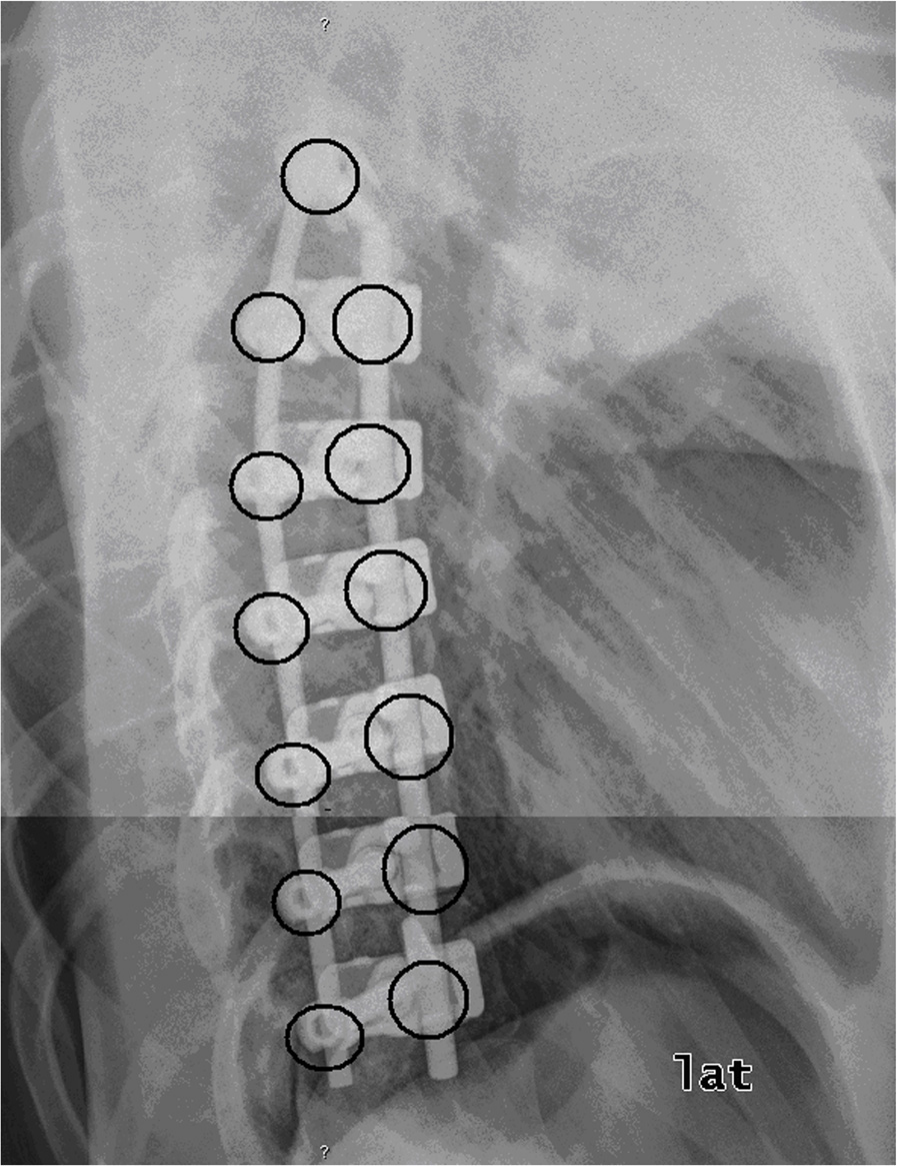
Technique for counting the different connections on a.p. radiographs of the spine.
